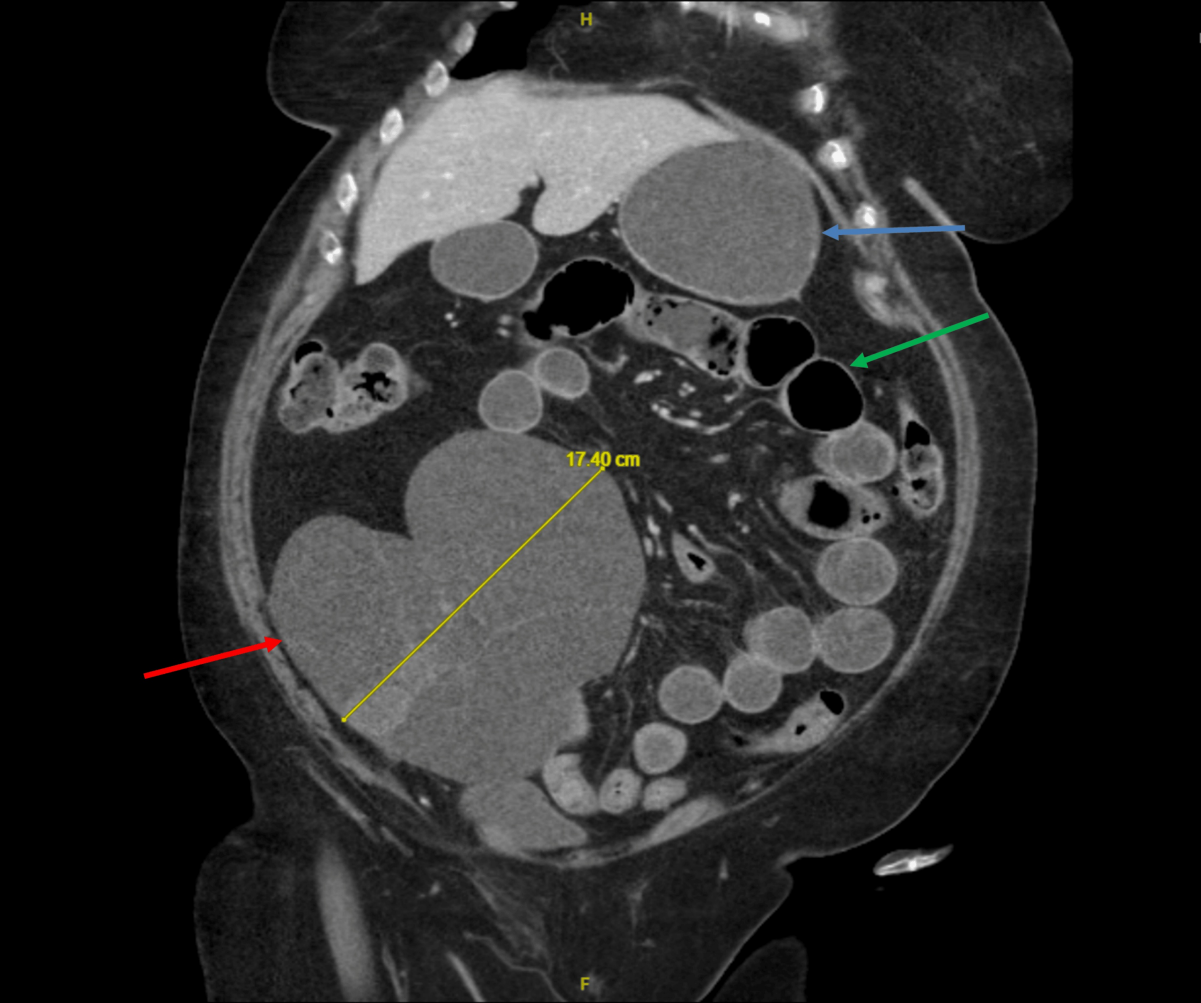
Contrast-enhanced CT scan in the coronal view reveals a large cystic mass (red arrow) present in the right lower quadrant of the abdomen measuring 17.4 cm wide. Fluid-filled stomach (blue arrow) and dilated bowel loops (green arrow).
